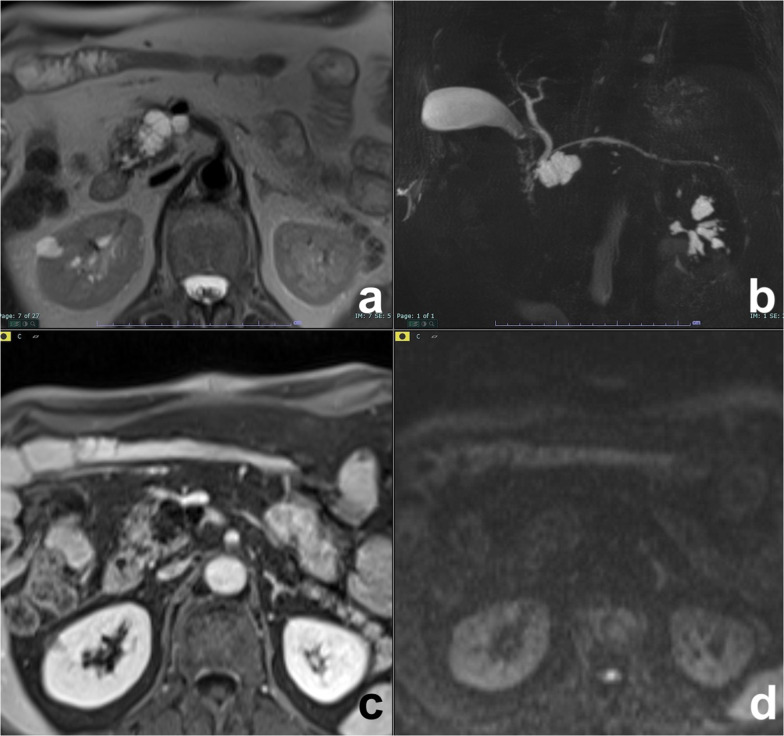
Unifocal BD-IPMN. On T2w (a) and MRCP (b), a cystic lesion with tiny septa is appreciable in the head of the pancreas. At T1w after paramagnetic contrast media injection (c), the septa show a slight enhancement. At DWI b 800 (d), no restriction diffusion can be observed
